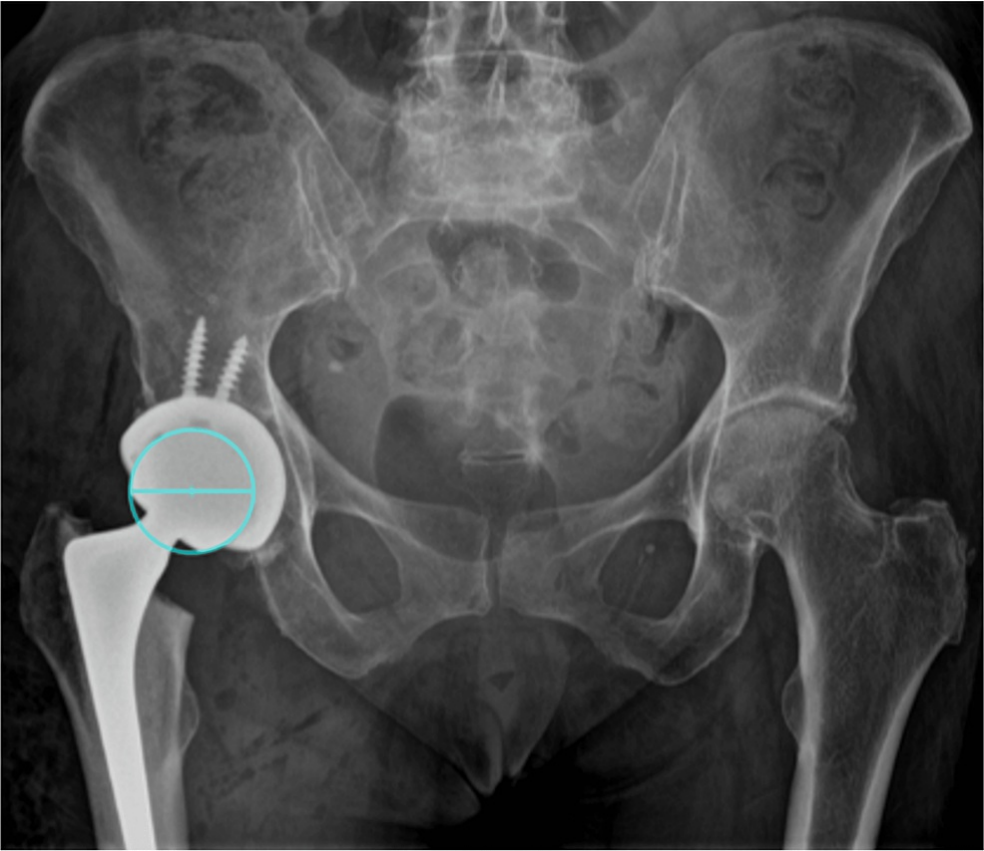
Post-operative anteroposterior pelvic radiograph visualised of a 45-year-old female on TraumaCad templating software for calibration of magnification using the known size of the femoral head.
